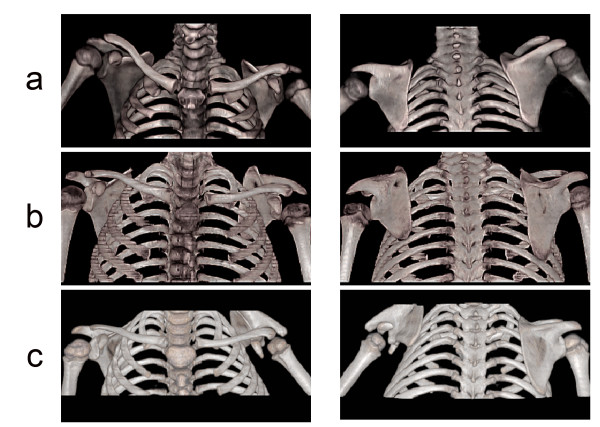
Three-dimensional CT Reconstructions Demonstrating Progressive SHEAR Deformities Misdiagnosed as Normal. Anterior (left) and posterior (right) three-dimensional CT reconstructions of bilateral CT scans of the upper extremities are depicted. Grade 2 (a), grade 3 (b), and grade 4 (c) SHEAR deformities are present in three OBPI patients that have 9.6%, 27.2% and 56.5% of the scapular body visible over the clavicle compared to the contralateral shoulder, respectively.
